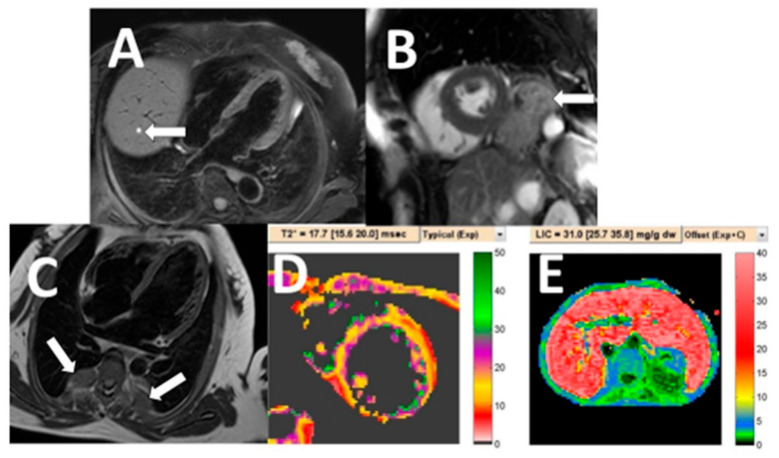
Examples of irrelevant non-cardiac abnormality on cardiac MRI. A cardiac MRI black blood image of a 52-year-old woman revealed a small, simple liver cyst (A: arrow). Cardiac MRI white blood image in short-axis plane detected hiatal hernia (B: arrow). Incidentally found extramedullary hematopoiesis (C: arrows) of thalassemia patient who underwent CMR for iron concentration quantification in the heart (D) and liver (E).
